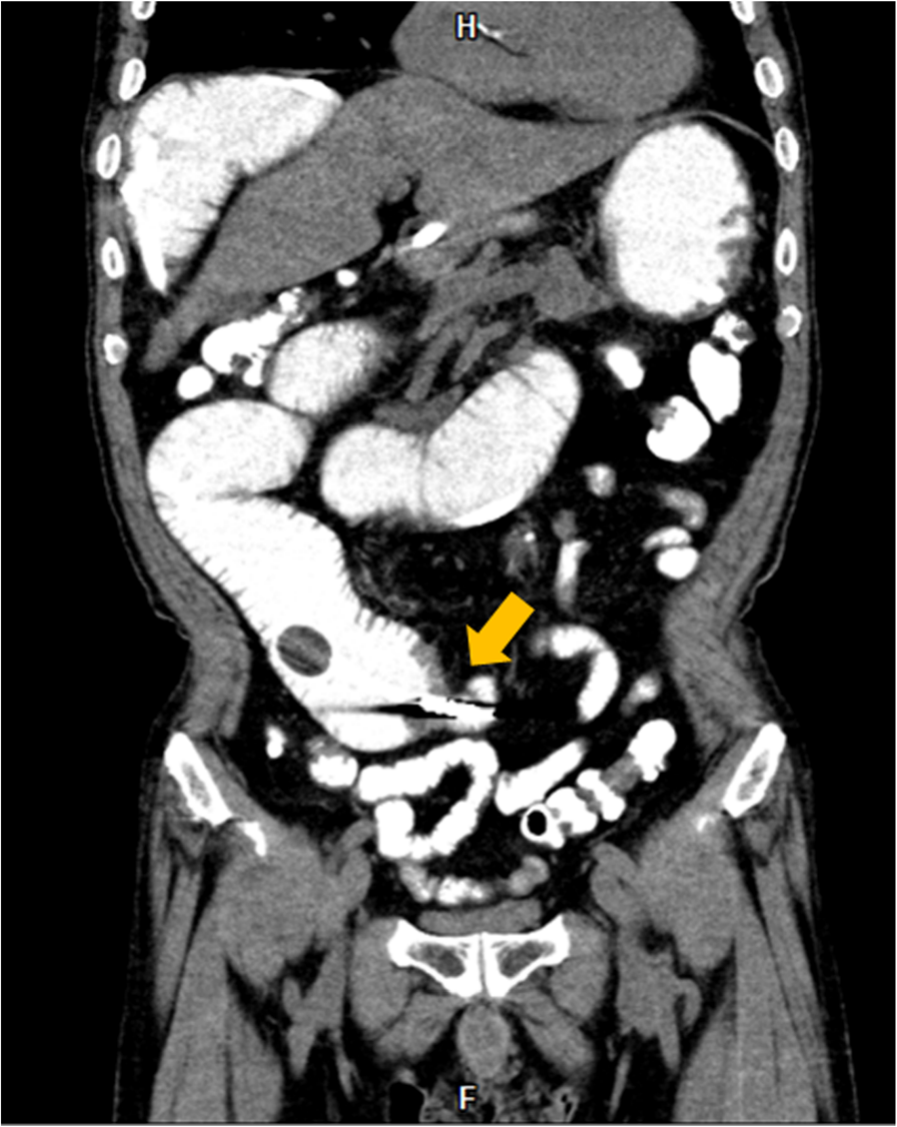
Computed tomography (CT) enterography. CT enterography suggested anastomotic stenosis, although water-soluble contrast medium passed through to the distal bowels. Arrow indicates caliber change at the jejunoileal anastomosis
